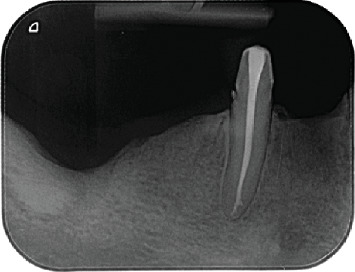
Intraoral periapical radiograph of the region 5 months postoperatively.
